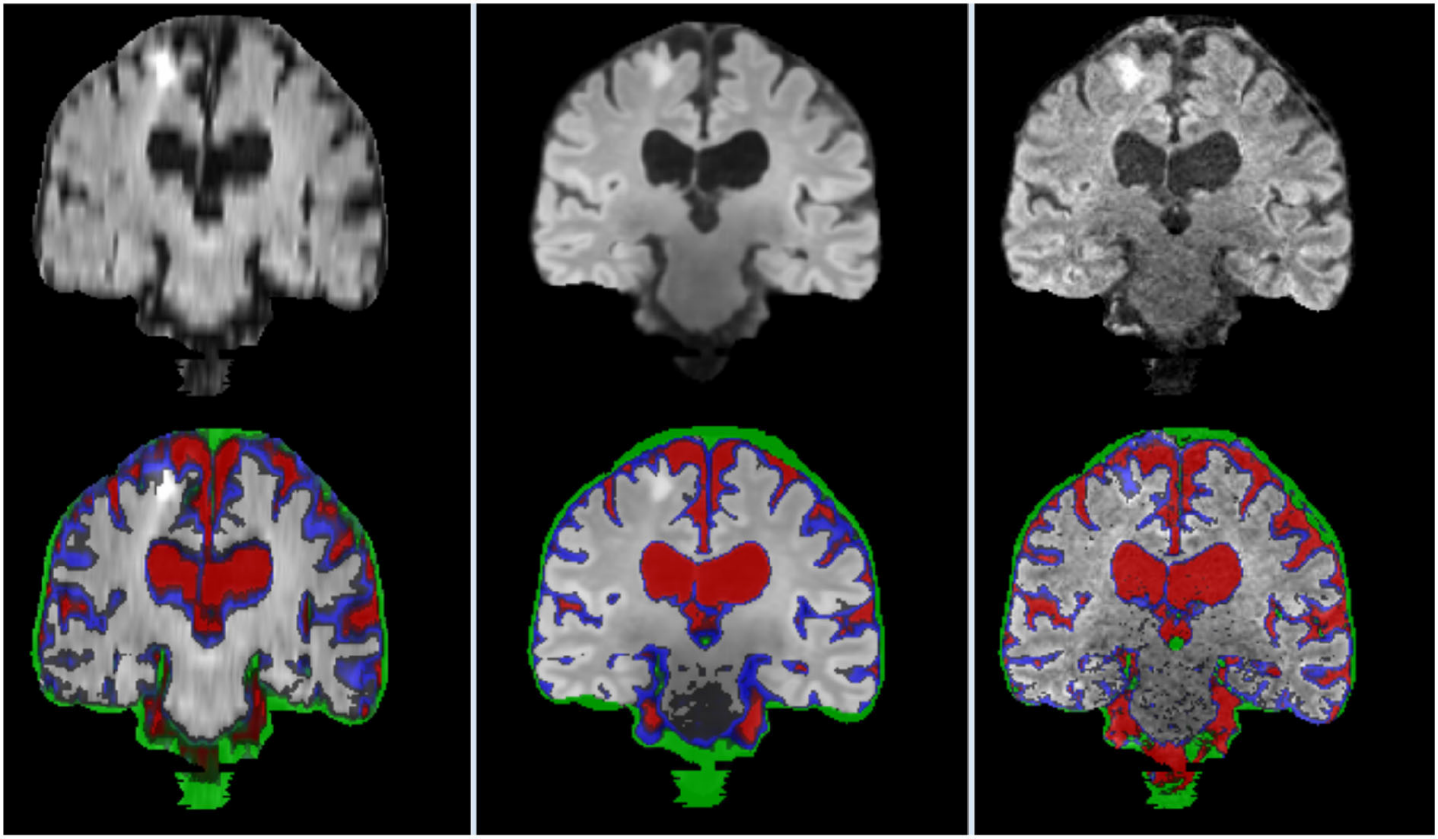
Coronal FLAIR slice of low resolution (left column) resampled and aligned to the high resolution image (right column) using trilinear interpolation, and the correspondent SR image (center column). Binary masks of CSF (red), pial layer (blue), and dural meninges and venous sinuses (green) are superimposed in the bottom row.
